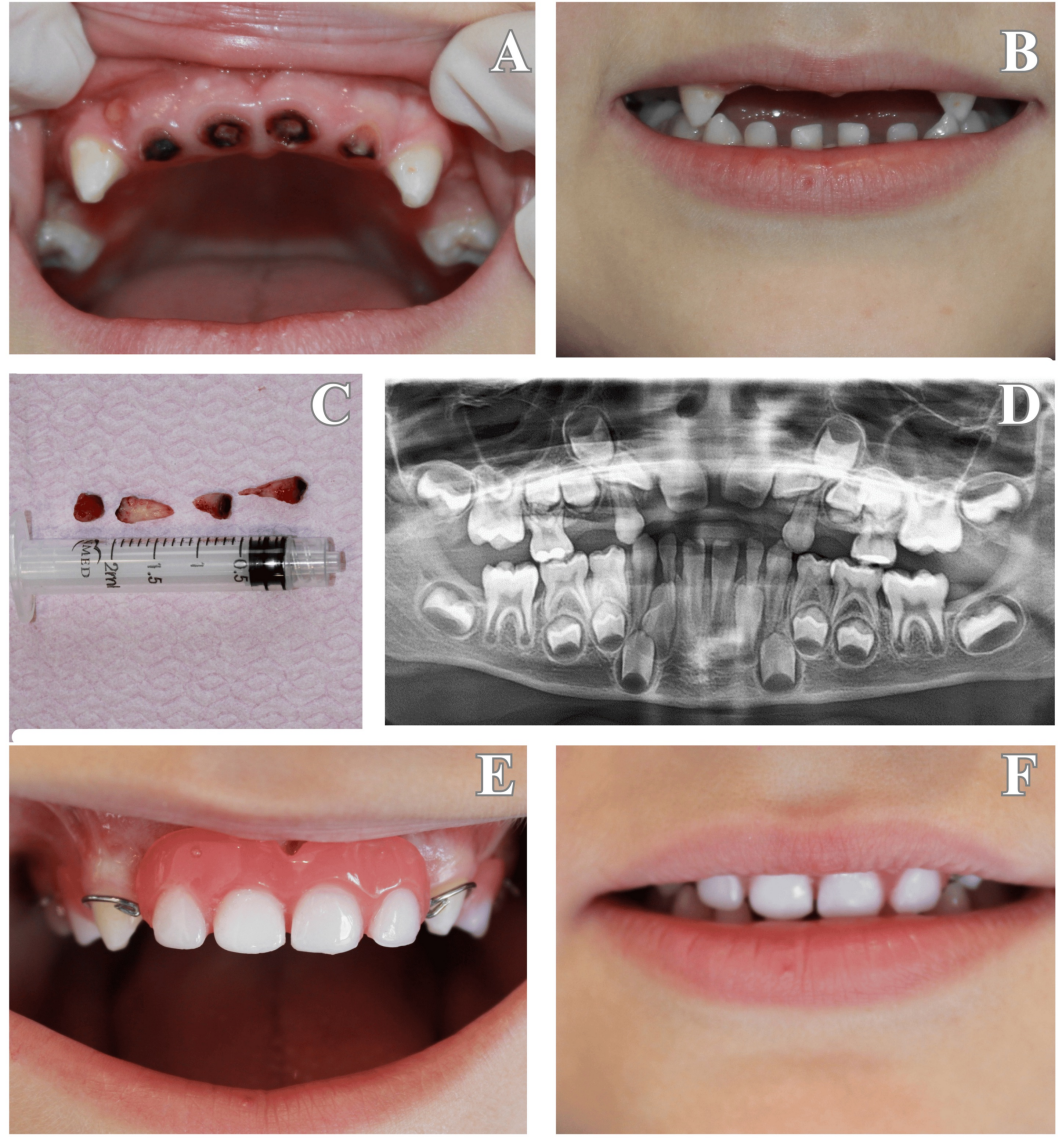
(A) Intraoral view showing extensive carious destruction and pulpal involvement of the maxillary primary incisors. (B) Preoperative frontal view of the patient demonstrating esthetic and functional compromise. (C) Extracted teeth (#52, 51, 61, 62, 54, and 64) due to severe caries-related complications. (D) Panoramic radiograph revealing multiple missing primary teeth and the stage of development of the permanent successors. (E) Intraoral view after the delivery of a removable functional space maintainer with esthetic anterior elements. (F) Extraoral view showing improved esthetics and smile harmony after appliance placement.
